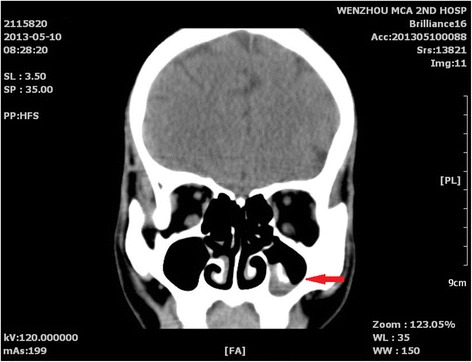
Coronal CT image of sinuses, indicating fungal rhinosinusitis of the left maxillary sinus
